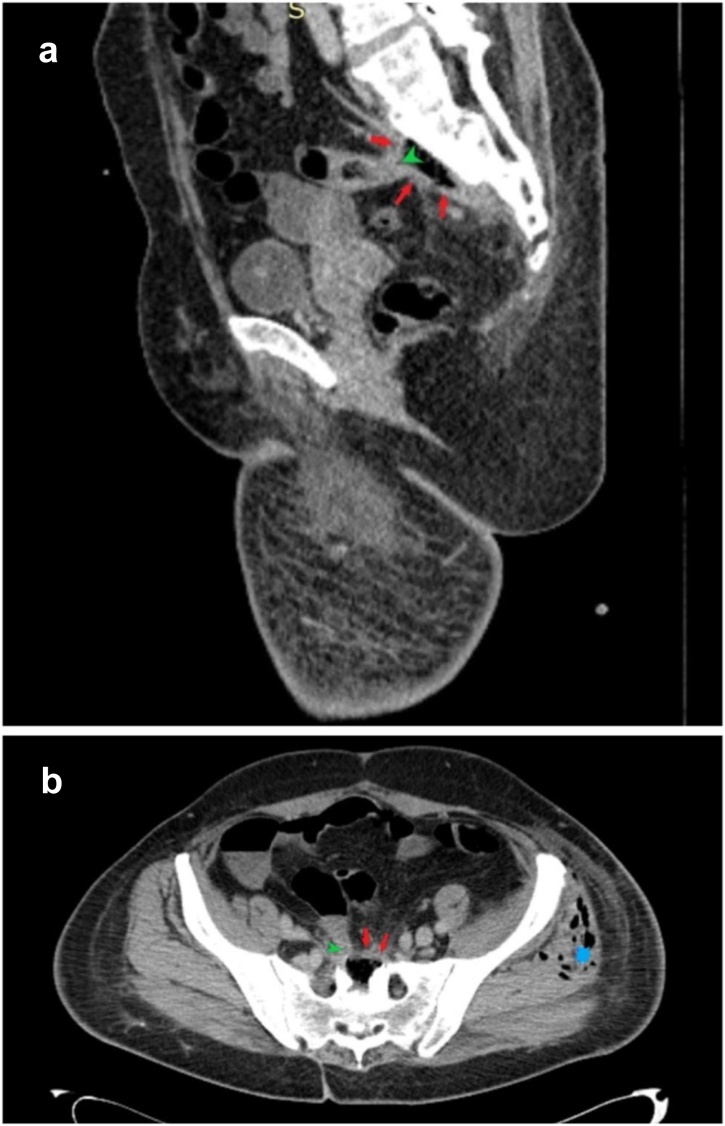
Sagittal (a) and axial (b) CT images of pelvis and presacral region. *Blue star* shows intramuscular gas regions (phlegmon). *Red arrows* mark presacral collection of gas interpreted as presacral abscess. *Green arrowheads* show ileal segment fixated to the anterior wall of presacral abscess, interpreted as fistula site.
